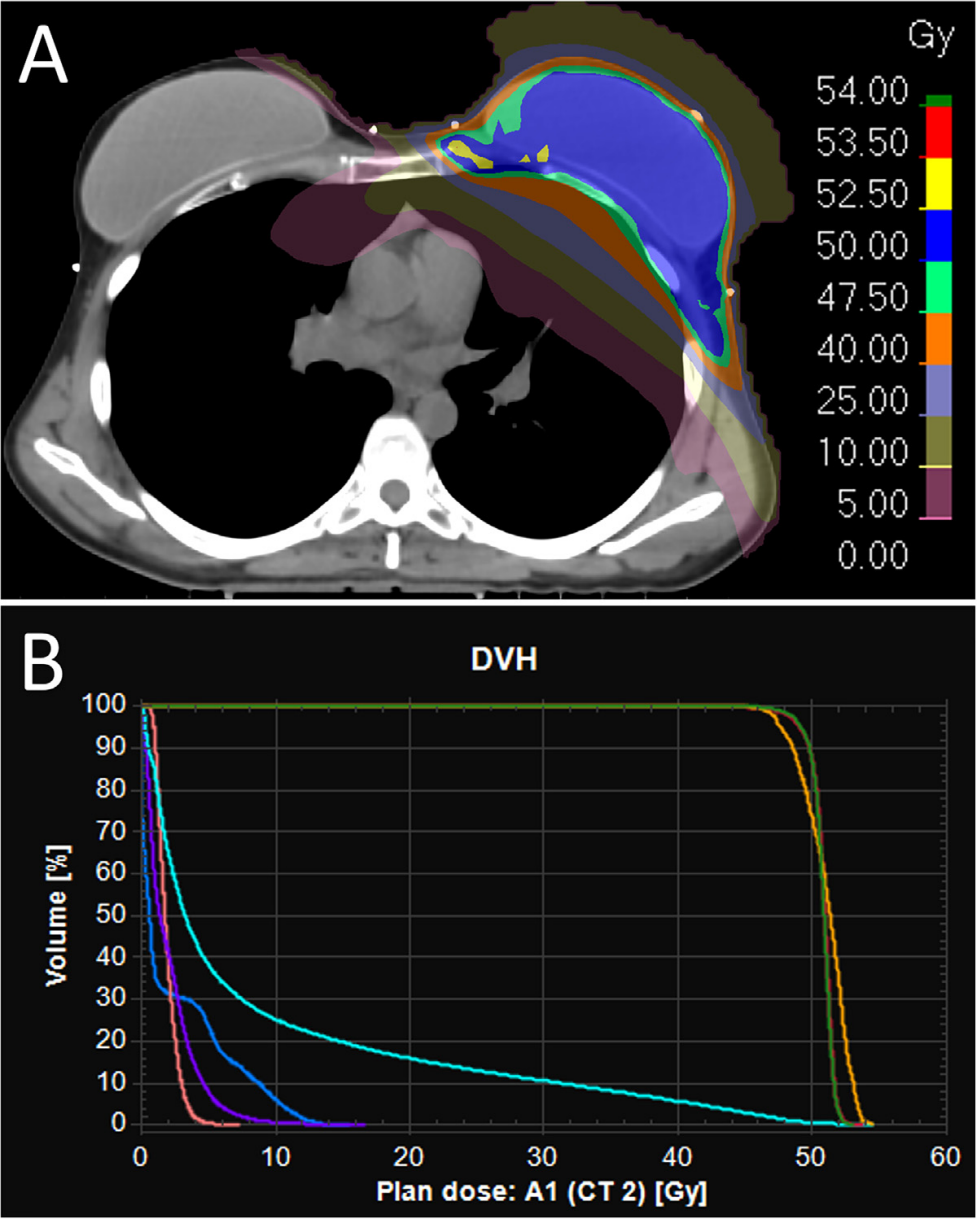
Radiation dose distribution on an axial computed tomography slice at the level of the reconstructed breast (A) and dose-volume histogram (B) of the presented patient's radiation therapy plan, the latter representing the volumetric radiation dose received by the breast planning target volume (red curve), axillary and supraclavicular planning target volume (green curve), internal mammary chain planned target volume (orange curve), left lung (cyan curve), spinal cord (blue curve), right lung (purple curve), and heart (pink curve).
